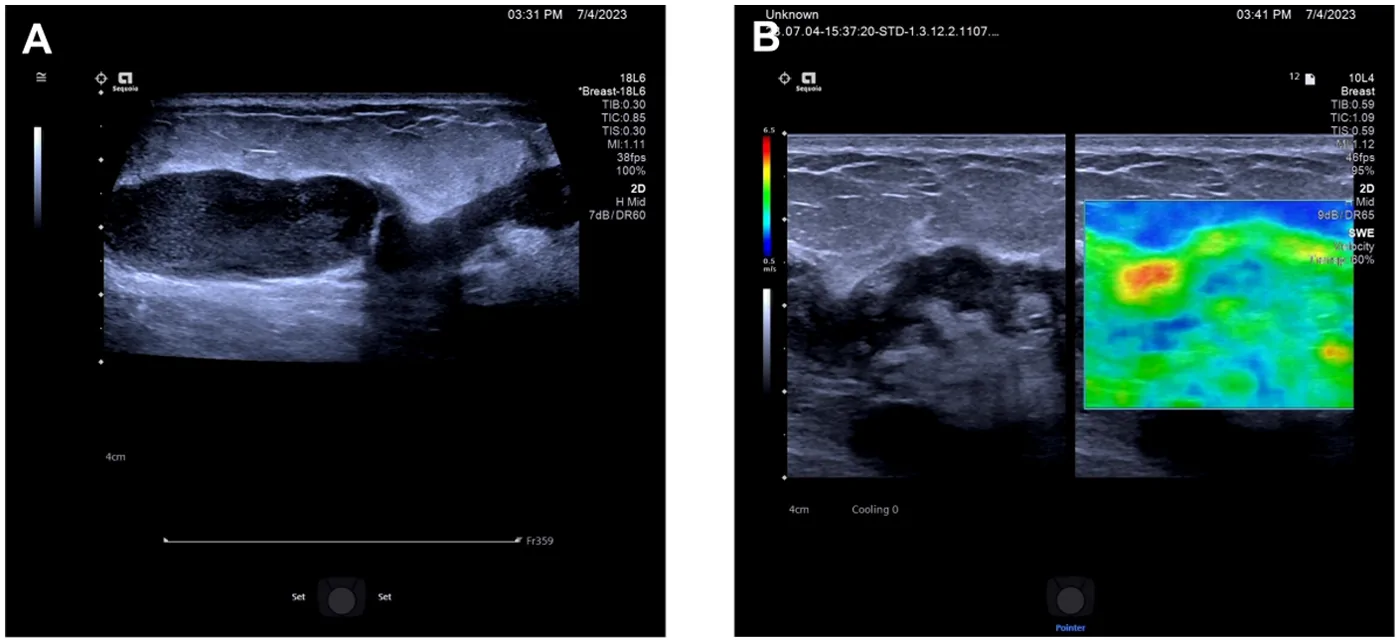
Preoperative ultrasonography of the breast. (A) Grayscale ultrasound showing a heterogeneous, predominantly hypoechoic lesion with irregular contours and internal echogenic material, suggestive of abscess formation in the clinical context. (B) Corresponding grayscale and elastographic images showing a heterogeneous elastographic pattern in the imaged region.
